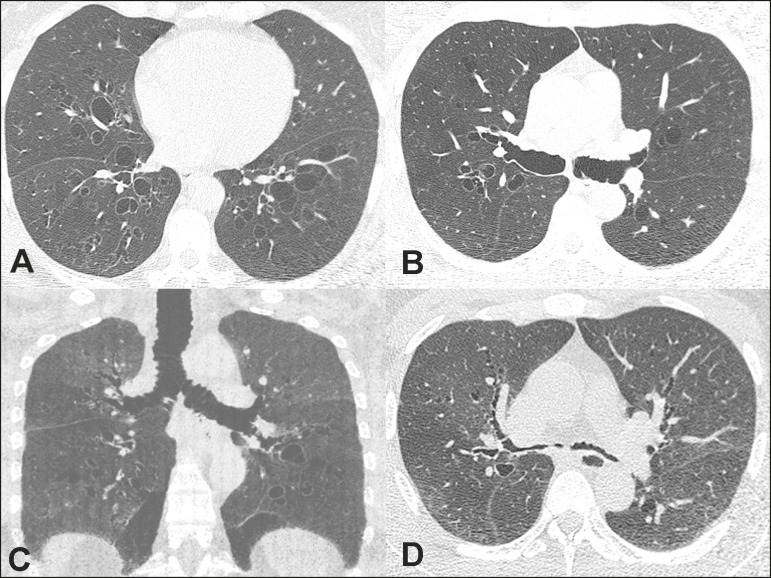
A,B: CT scan of the chest, acquired during inspiration, showing
